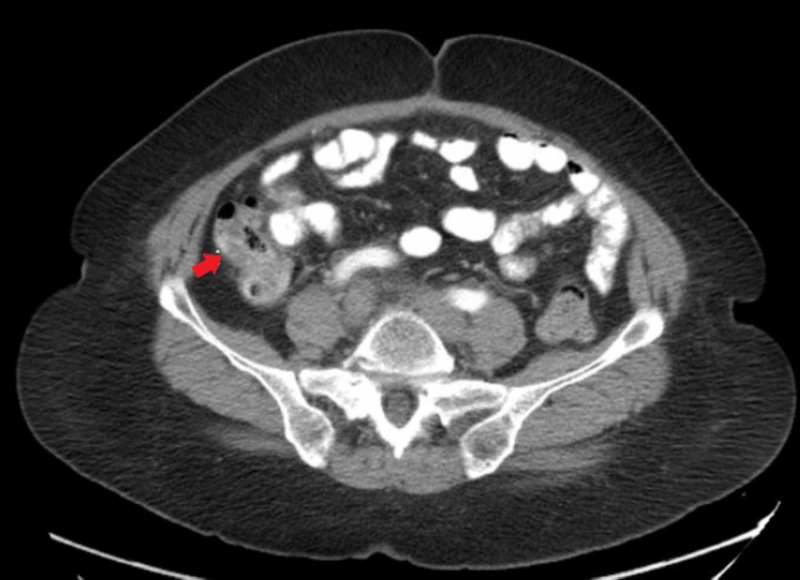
CT abdomen and pelvis with contrast.
